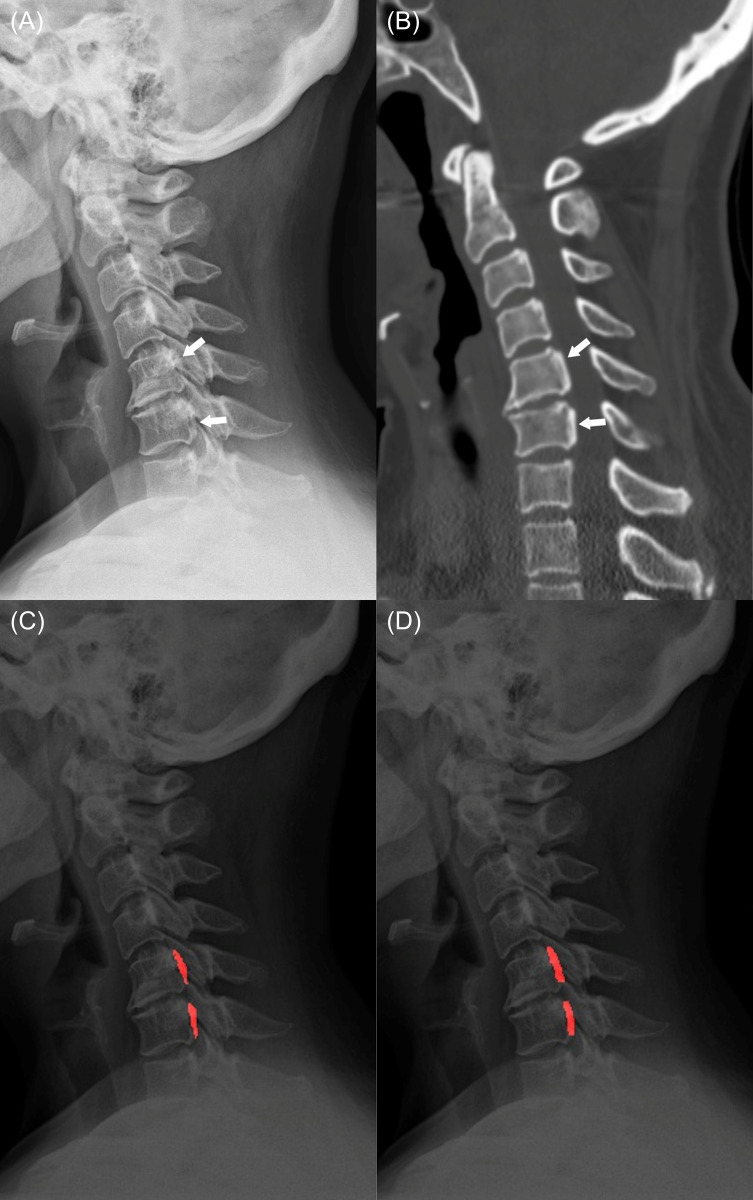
A 51-year-old female patient with segmental-type OPLL. (a) A lateral plain radiograph shows segmental-type OPLL (arrow) at the C5-6 level. (b) A sagittal image of cervical spine CT also demonstrates the segmental ossifications (arrow) at C5-6 level (window width = 2000 HU, window level = 500 HU). (c) OPLL lesions annotated by a radiologist on the plain radiograph. (d) The deep-learning model correctly predicted the segmental-type OPLL, which was overlooked by two observers. OPLL, ossification of the posterior longitudinal ligament.
